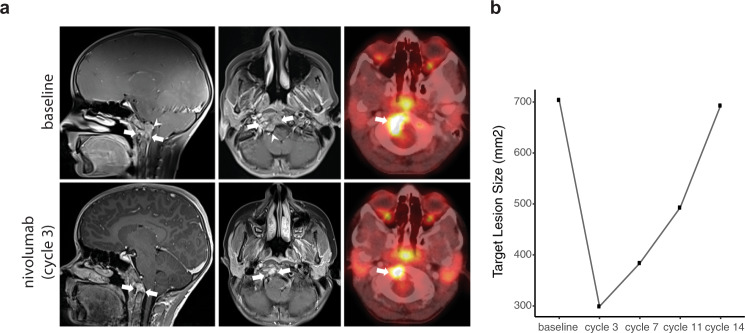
Response of a poorly differentiated paediatric chordoma to nivolumab. a Sagittal (left) and axial (middle) post-contrast MRI and concurrent PET/CT (right) images prior to nivolumab therapy (top panel) demonstrate an enhancing solid mass involving the clivus, basiocciput and right occipital condyle (arrows), with effacement of the premedullary cistern and indentation upon the brainstem (arrowheads) and a large focus of metabolic activity at the tumour site (SUVmax 8.2; arrow). Follow-up images (lower panels) 3 months after the initiation of nivolumab therapy show substantial decrease in size of the mass (arrows), with resolution of mass effect upon the brainstem. The concurrent PET/CT image shows attendant decrease in the region of metabolic activity (SUVmax 6.0; arrow), indicating a partial radiographic response. b Target lesion size at baseline and following 3, 7, 11 and 14 cycles of nivolumab.
